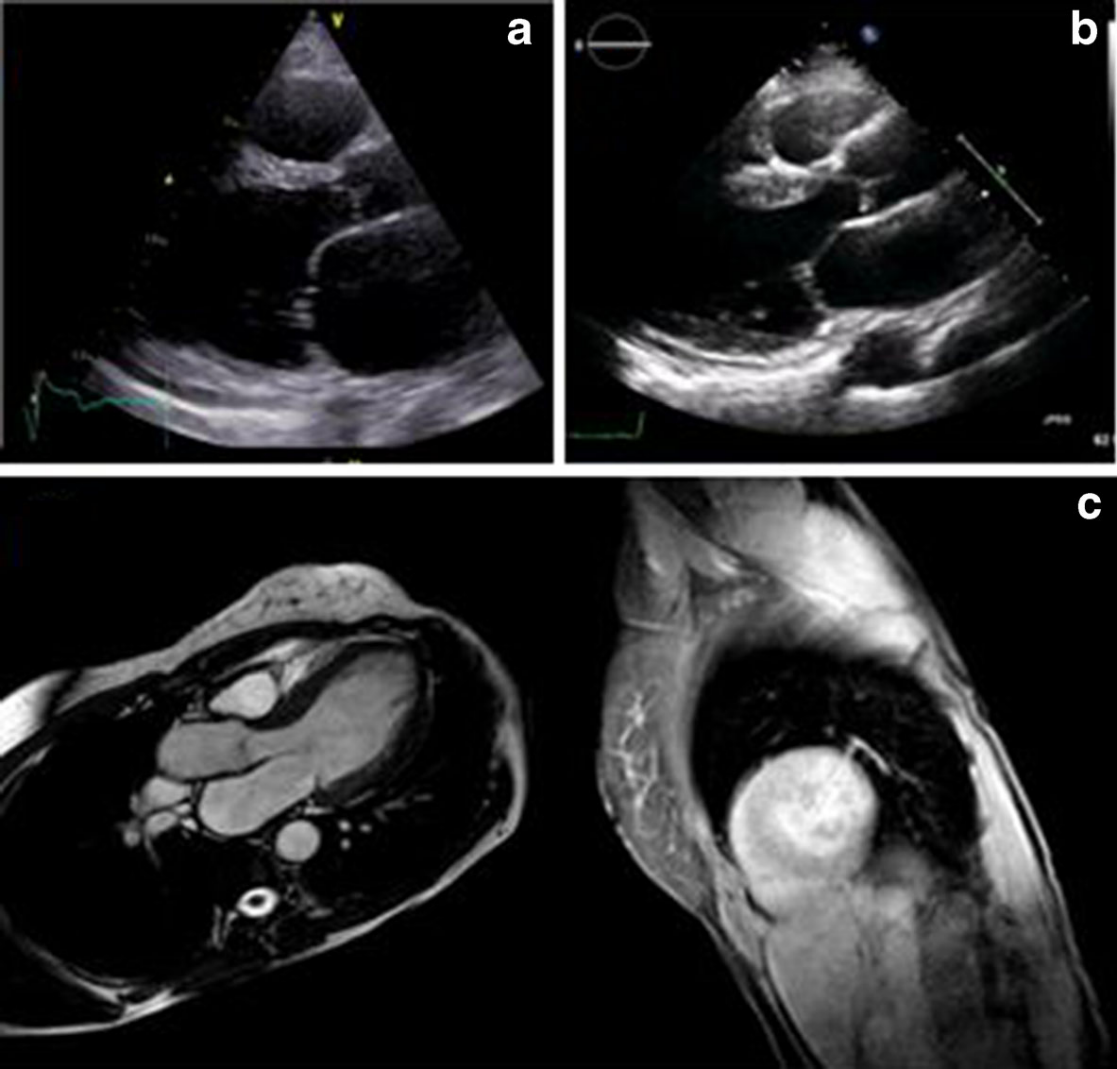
Echocardiograms from the two patients presented. Panel a, parasternal images from the asymptomatic daughter. The interventricular septum measured 15 mm (reference < 11 mm). Panel b, the echocardiogram from the mother aged 81 years. The scan demonstrates a dilated left ventricle (LVIDd 46 mm/m^2^, reference < 32 mm/m^2^) and severe systolic heart failure (left ventricular ejection fraction estimated to 15%). Panel c, cardiac magnetic resonance imaging of the daughter. The cardiac magnetic resonance scan demonstrated preserved systolic function and mild left ventricular hypertrophy (143 g/m^2^, normal range 69–141 g/m^2^). The cardiac magnetic resonance also demonstrated late gadolinium hyperenhancement in inferolateral left ventricular myocardial segments, that is, in a pattern highly suspicious of a cardiac phenotype with Fabry’s disease
